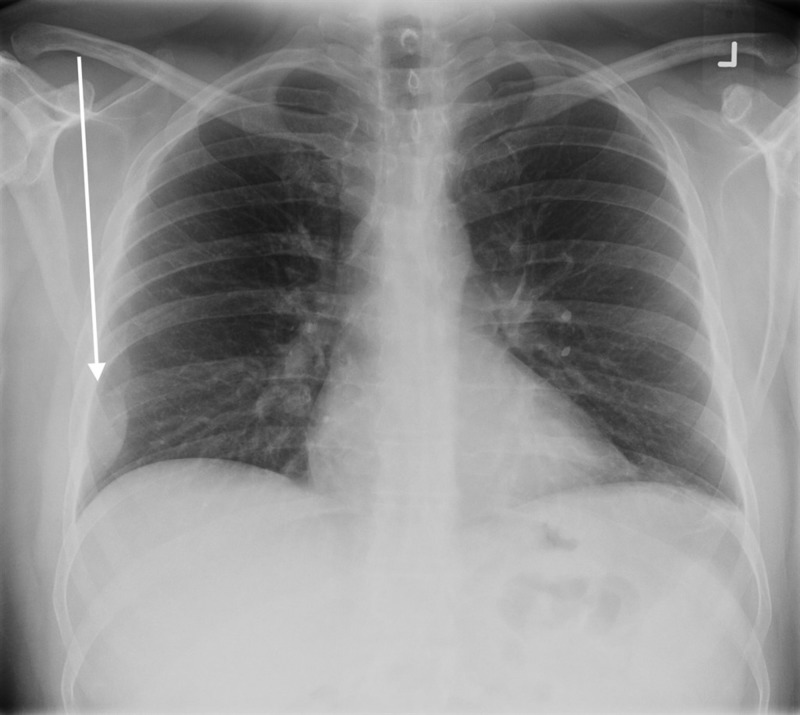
Pleural-based opacity identified within the right lower hemithorax. No rib fractures were identified. CT was recommended to evaluate the pleural lesion. CT: computed tomography
